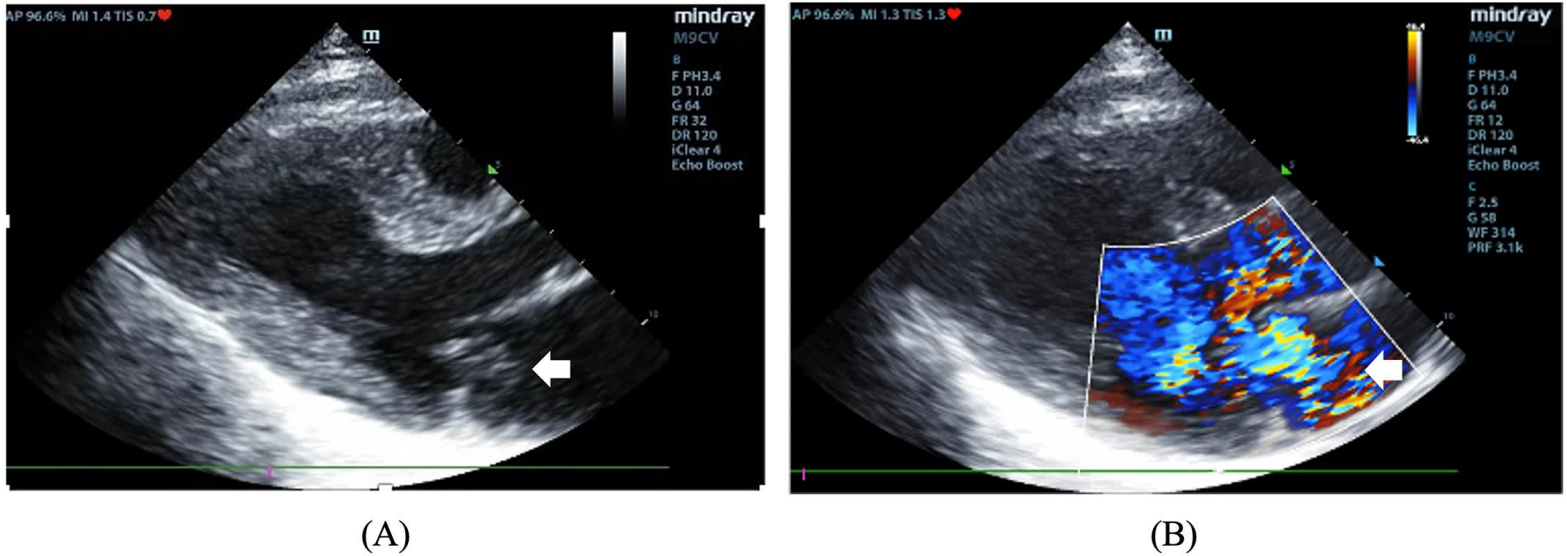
(A) The two-dimensional image showing a mass protruding into the left atrium (arrow). (B) Color Doppler image showing severe mitral regurgitation.
